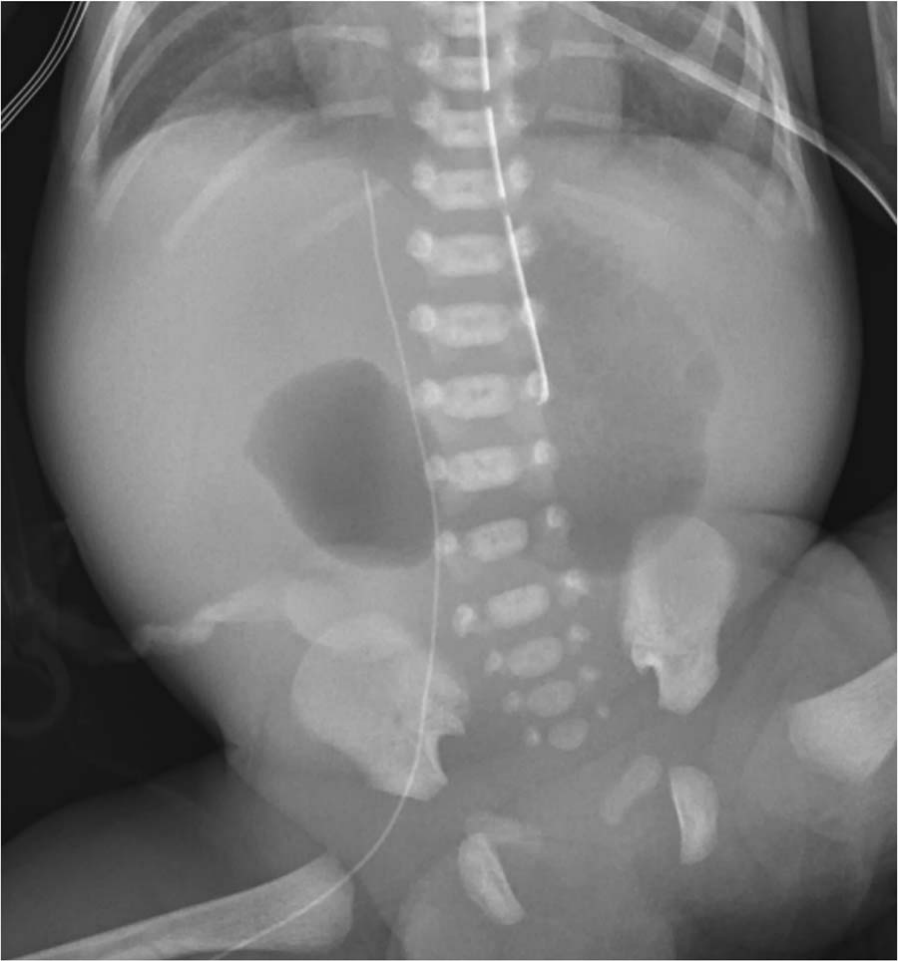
“Double bubble” sign on X-ray.
